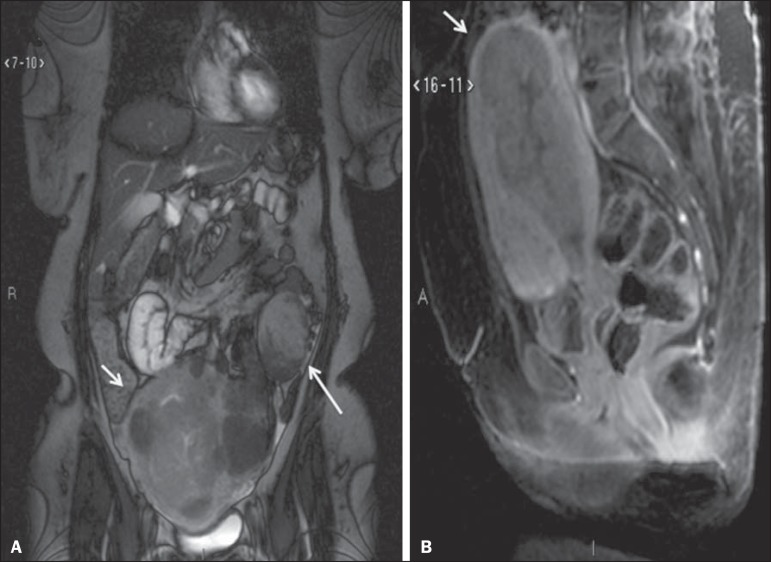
MRI scan requested for the diagnosis of an adnexal mass.
